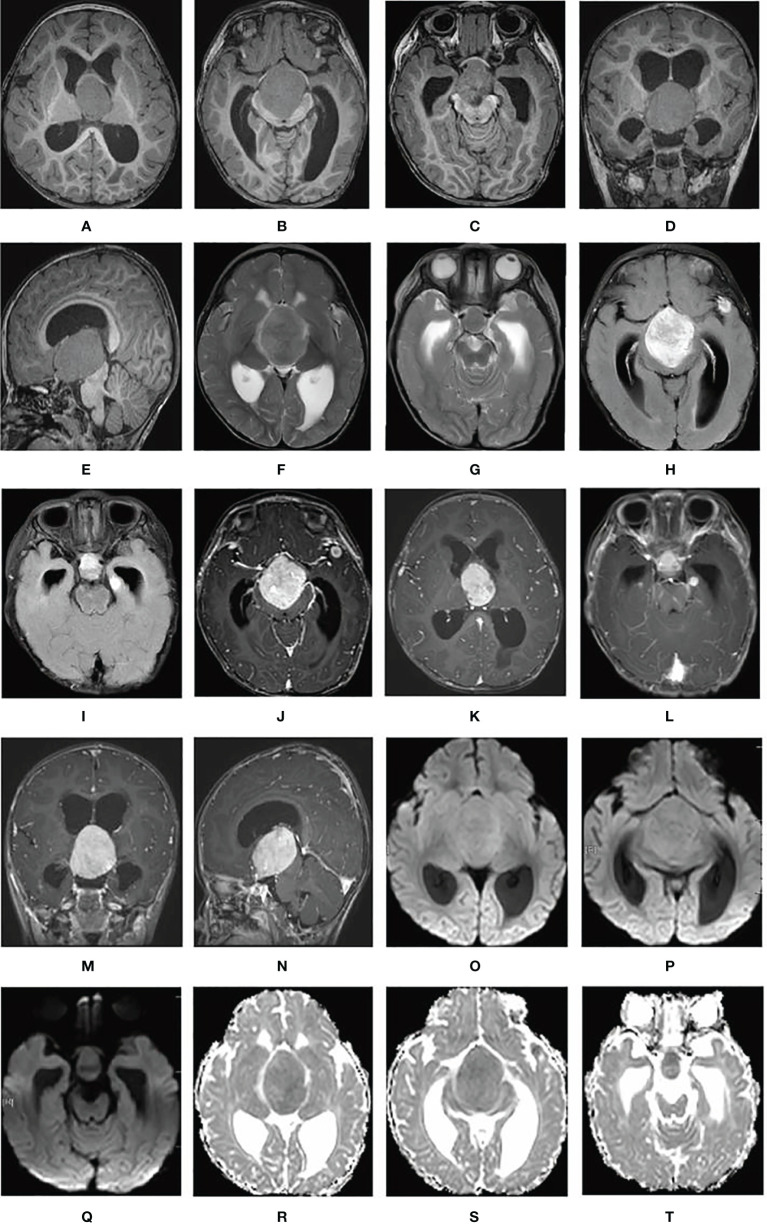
Cerebral lesions are seen on MRI. (A–E) T1-weighted MRI; (F, G) T2-weighted MRI; (H, I) fluid-attenuated inversion recovery MRI; (J–N) contrast-enhanced T1-weighted MRI; (O–Q) diffusion-weighted imaging; and (R–T) apparent diffusion coefficient.
